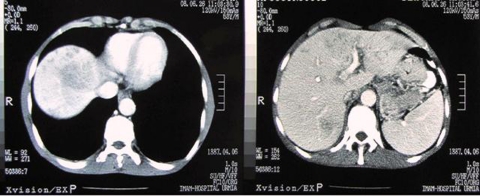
Note the mass lesion in the right liver lobe on abdominal CT performed with intravenous contrast
